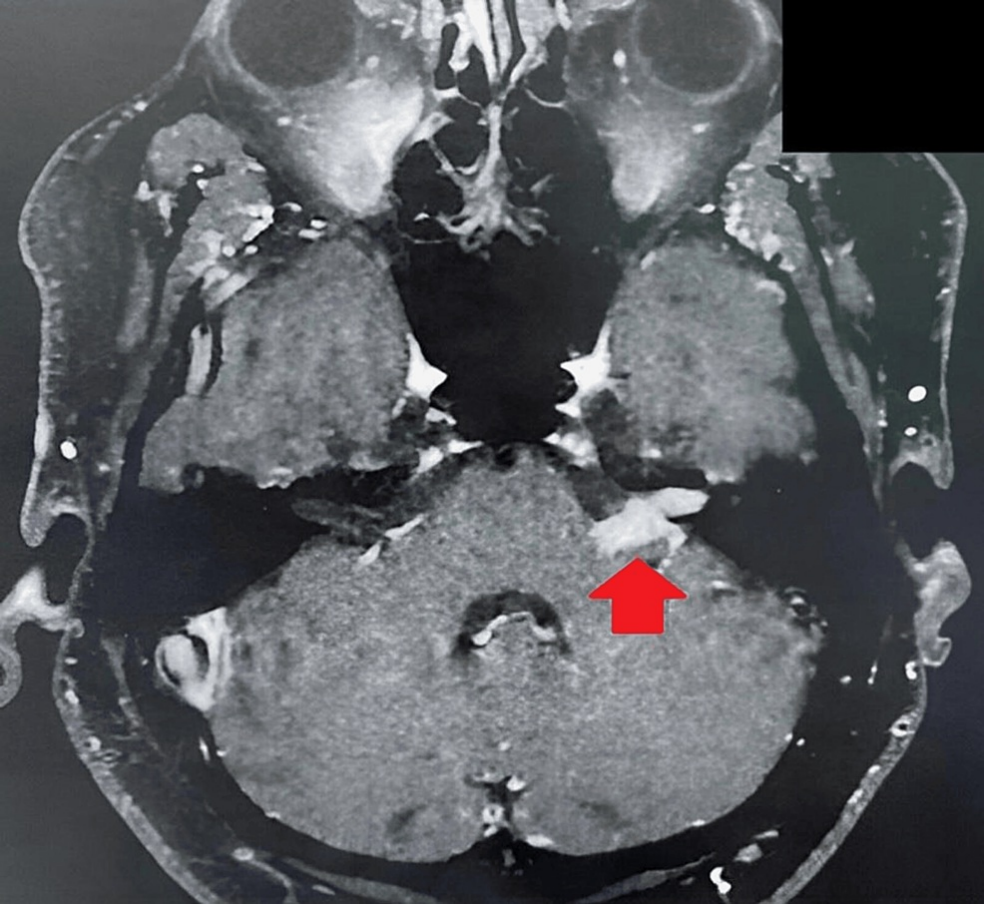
Preoperative MRI on T1 showing an expansive lesion with enhancement after gadolinium administration located along the pathway of the left internal auditory canal and cerebellopontine angle, measuring approximately 1.4 cm on the laterolateral axis. The red arrow shows the tumor.
